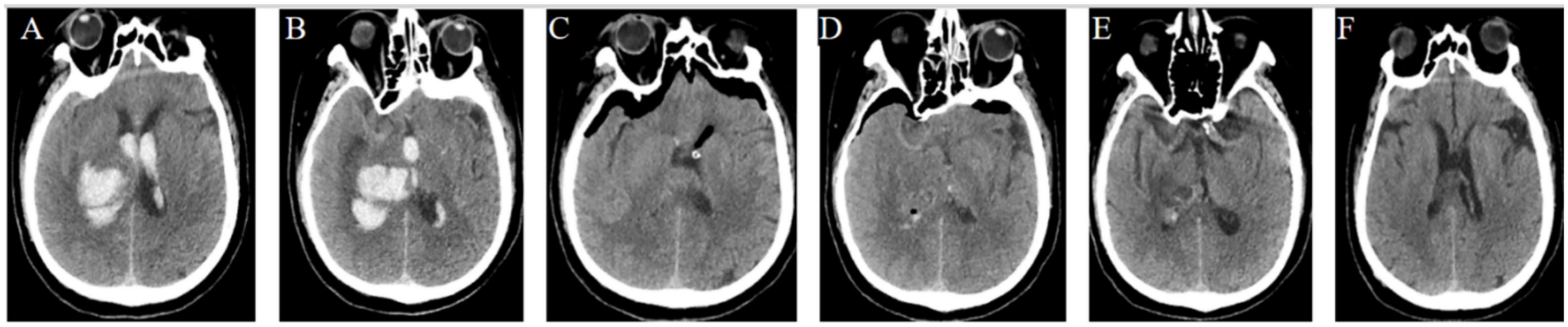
Pre- and post-operation CT scans of an irregular thalamic hemorrhage breaking into the ventricles that was evacuated by FATSE combined with a “Two-in-One” neuroendoscopic approach. (A) A CT scan collected upon admission showing a hematoma in the right thalamus and the expansion of the hematoma that broke into the lateral ventricles. (B) The CT scan showing hematoma extension into the third ventricles. (C) Postoperative CT scan showing that the hematoma in the thalamus and lateral ventricles almost completely evacuated. (D) CT scan showing hematoma in the third ventricles were removed successfully. (E) Postoperative 2 weeks CT image showing good results. (F) CT image showing no hydrocephalus after 2 weeks.
